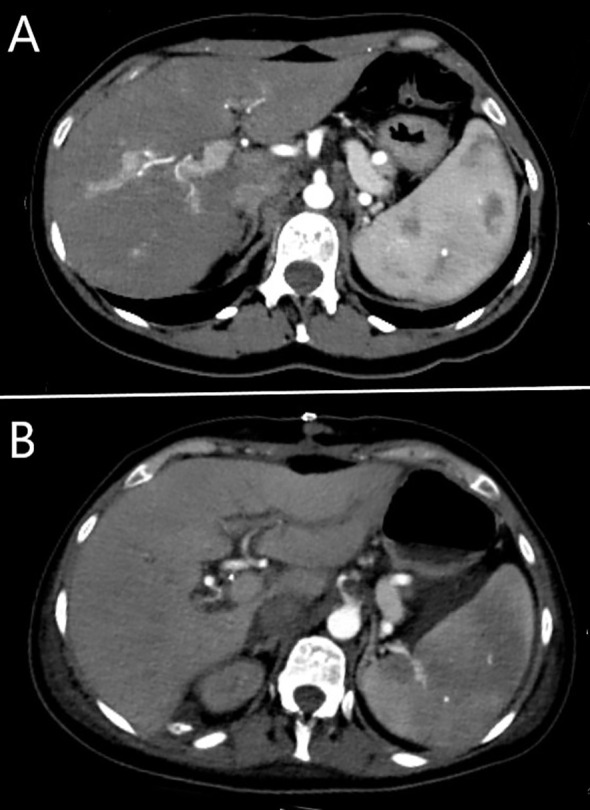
Preoperative computed tomography of the abdomen two weeks after admission to the hospital and occurrence of acute liver injury (A). Postoperative computed tomography of the abdomen after LT (B).
